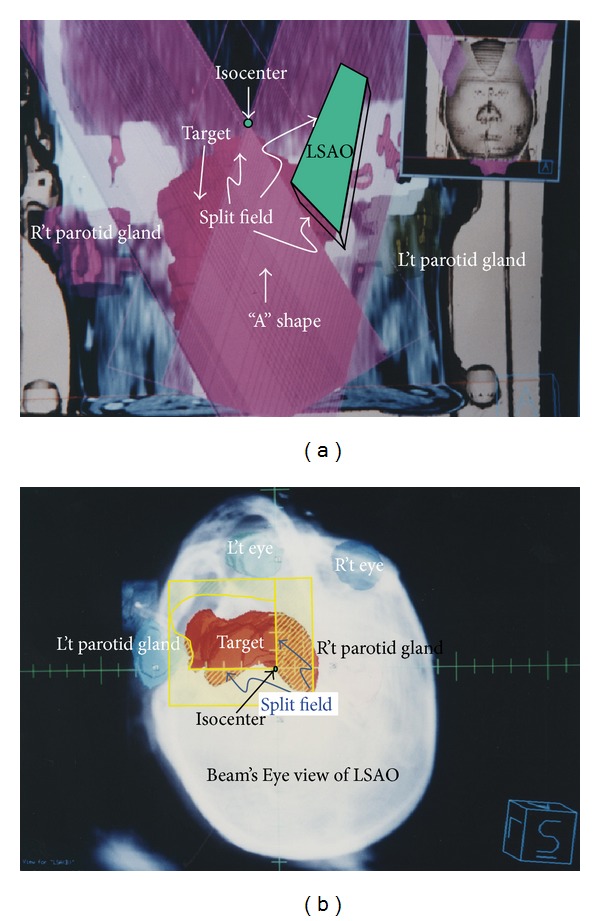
(L't) Left superior anterior oblique (LSAO) field was added to create an “A” shape dose overlap region (overlapping of RSAO and LSAO). This picture shows the split field of LSAO in coronal plane. Right and left side parotid glands were represented in pink and brown colors, respectively. In this field, couch was rotated to 250°, gantry was rotated to 98°, and collimator was rotated at degree of 172°. Sixty-degree wedge with “thick head” toward gantry side was added for dose uniform modification. (R't) This picture shows the Beam's Eye View (BEV) in Digital Reconstruction Radiograph (DRR) of LSAO field. Two central axis nondivergence planes (denoted as “split field” on the left hand side) are ready to be abutted by RAO (vertical) and SIV (horizontal) fields.
